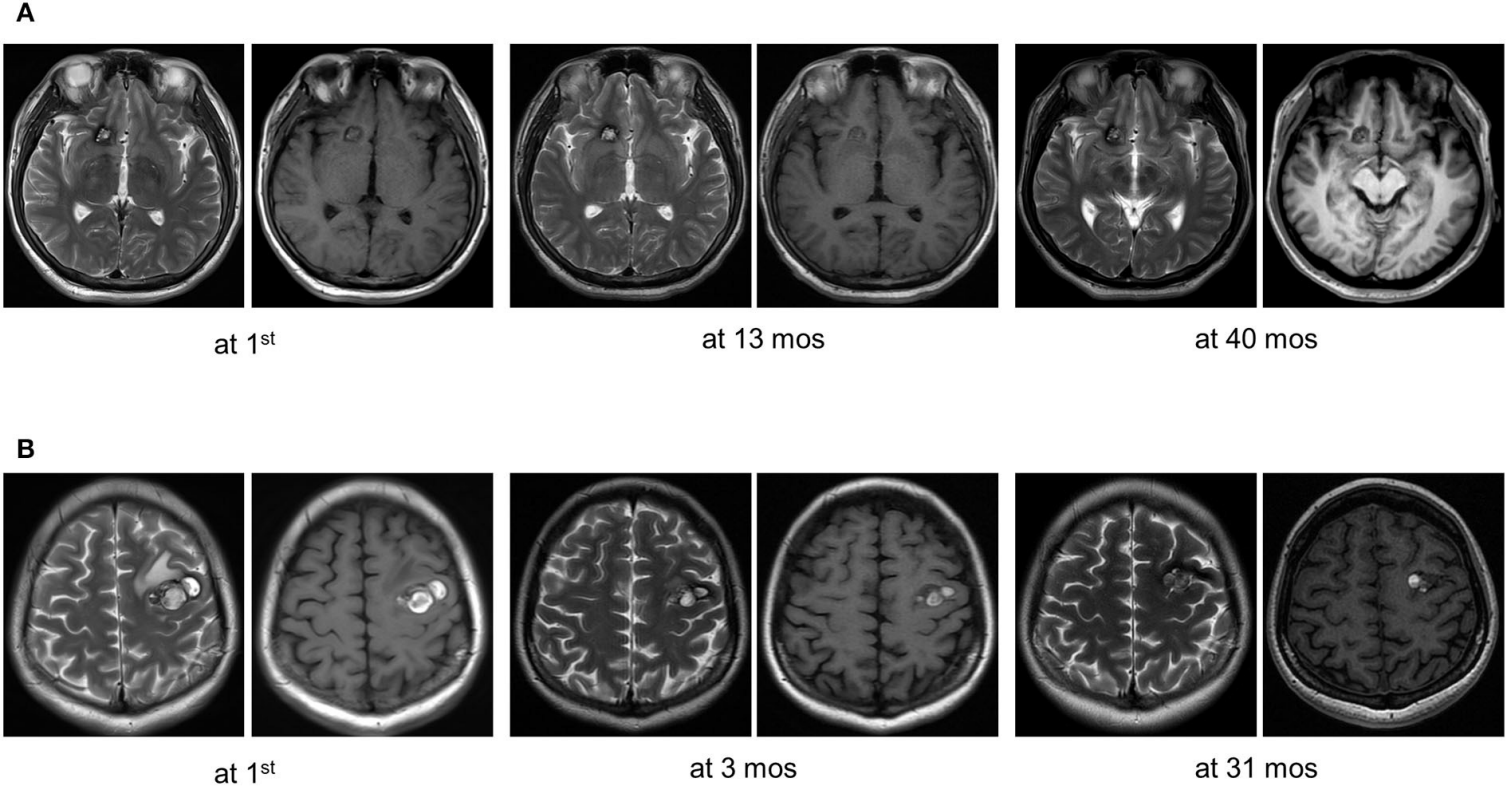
Representative follow-up MRI scans of *MAP3K3* mutation and CCM gene mutation lesions before surgical resection. (A) A patient who harbored a *MAP3K3* mutation lesion remained stable based on follow-up MRI scans at 13 and 40 months. (B) A patient who harbored a *CCM1* mutation lesion exhibited recurrent overt hemorrhage based on follow-up MRI scans at 3 and 31 months.
